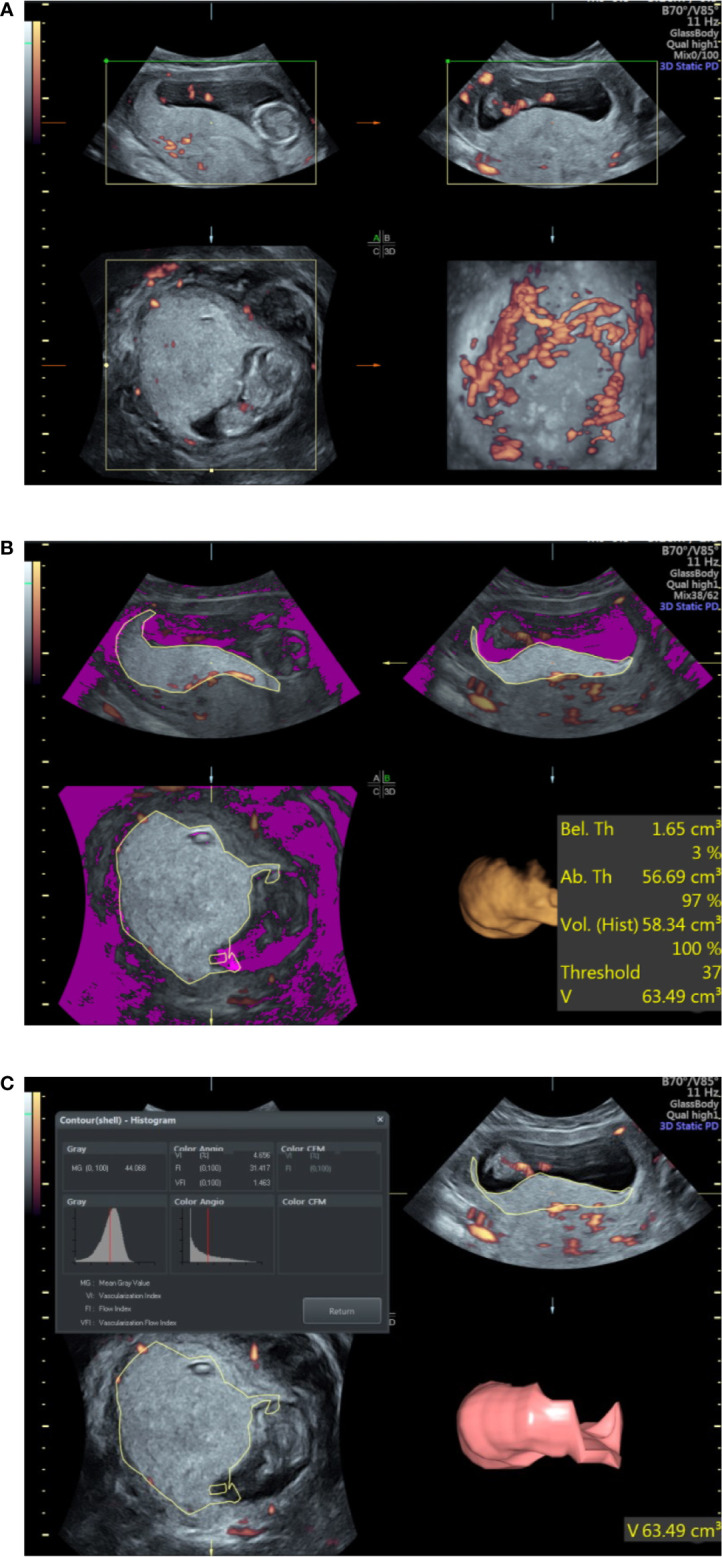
Ultrasound examination of the placental volume and vascular perfusion. (A) Placental volume as determined by three-dimensional power Doppler. (B) Measurement of placental volume by VOCAL. (C) Placental vascular indices determined by three-dimensional power Doppler.
